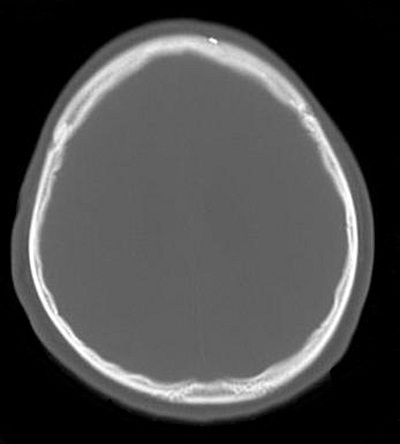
Bone window of cranial CT scan showing shrapnel in left frontal bone (same side as thalamic abscess)
